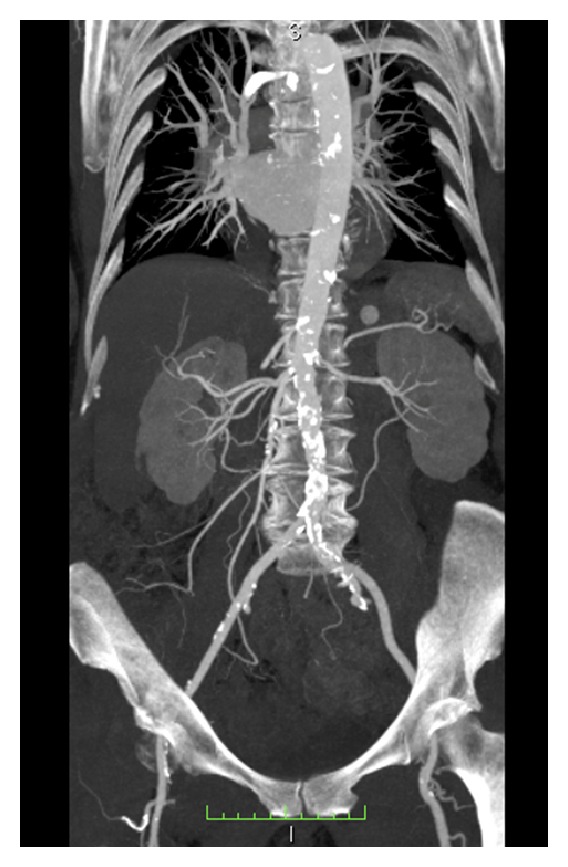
Aortoiliac CT Angiography for planning of transcatheter aortic valve implantation (TAVI), using high-pitch 128-dual source CT*. Severe calcifications of the abdominal aorta but the right iliac artery are spared from atherosclerosis and do not show tortousity. Transfemoral access was possible via the right iliac artery.
